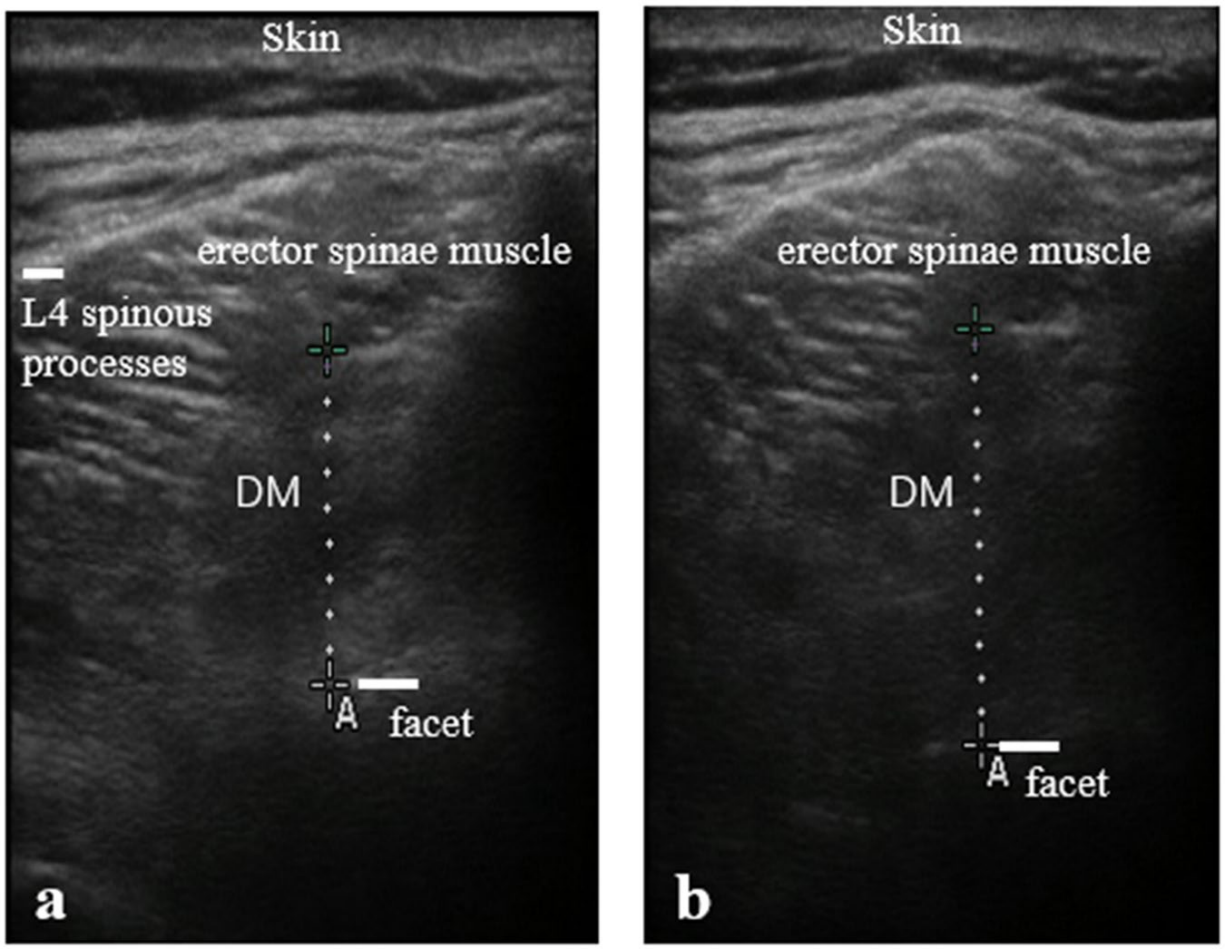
Representative ultrasound images of the deep multifidus (DM) from a patient with chronic low back pain (sagittal plane at L4 level) at rest (a) and during isometric activation (b). The line segments for labeling denotes the measure between the inferior border of the erector spinae muscle and the superior tip of the facet joints. L4, fourth lumbar vertebra.
